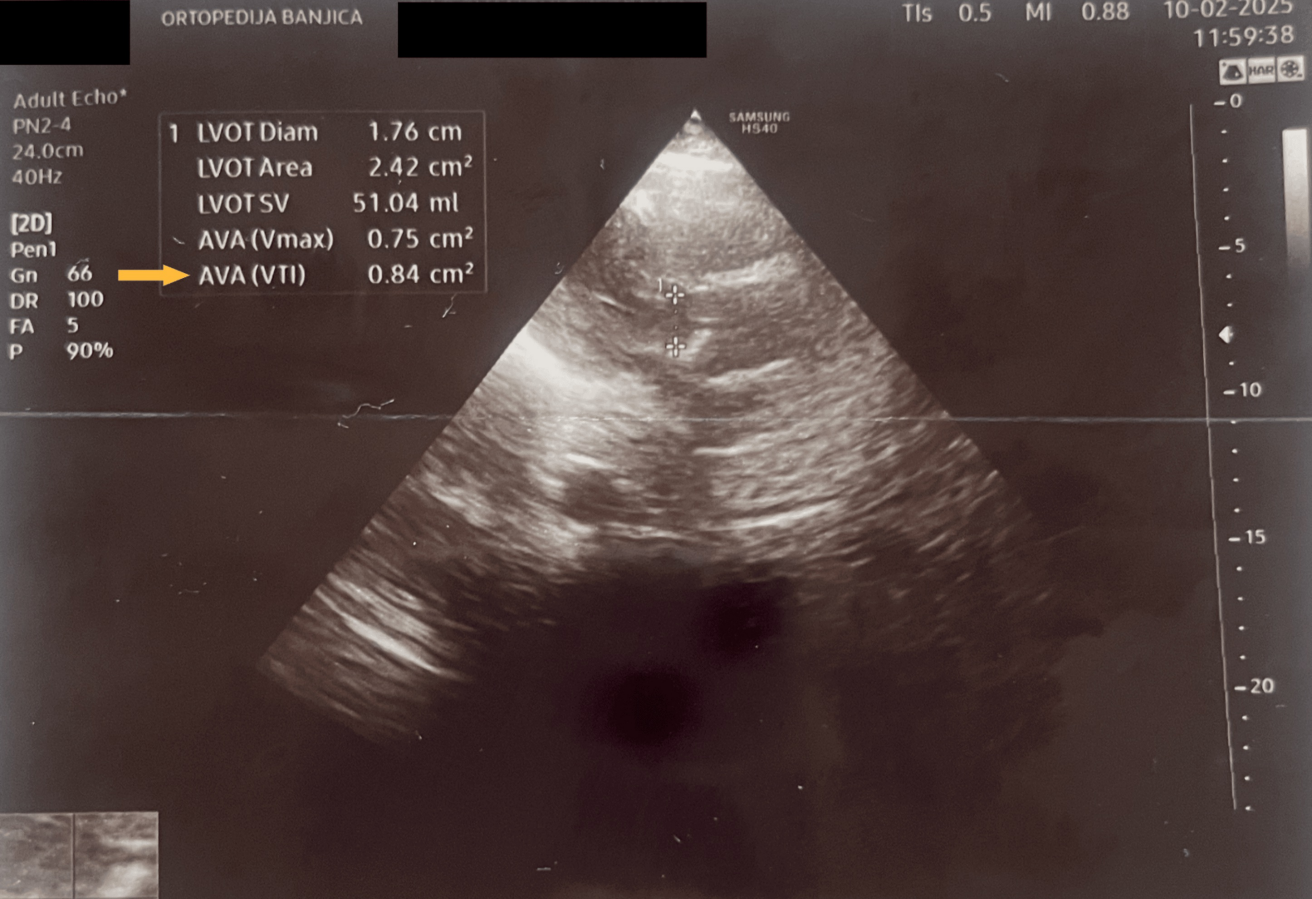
Echocardiographic image showing the aortic valve On echocardiography, aortic stenosis was confirmed; the yellow arrow indicates the measured aortic valve area (AVA), which is 0.84 cm^2^.
